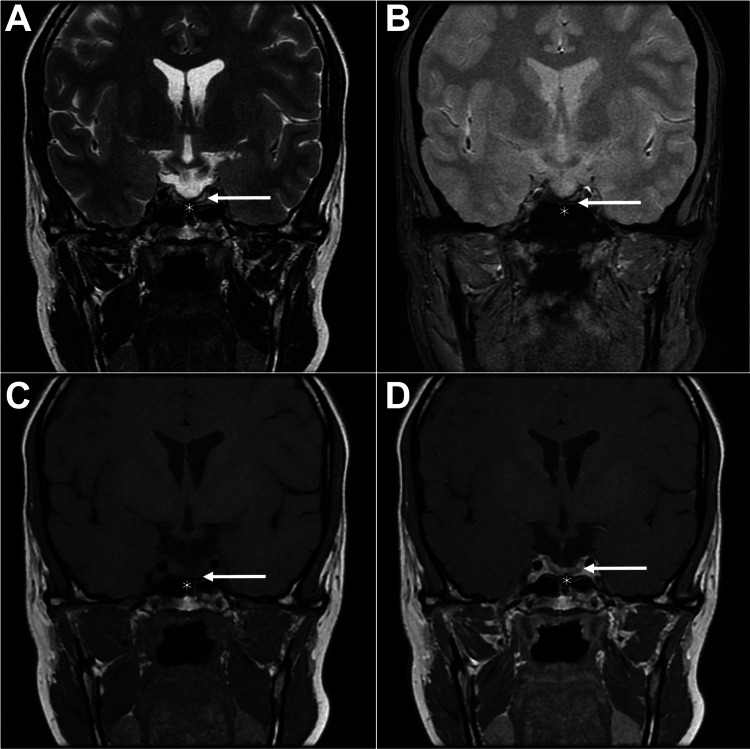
One-year postoperative follow-up MRI after transsphenoidal surgery Coronal T2-weighted (A), coronal T2*-weighted (B), coronal T1-weighted (C), and post-contrast coronal T1-weighted (D) images demonstrate postoperative changes consistent with a transsphenoidal approach, including sellar floor dehiscence (asterisk). The sella is filled with cerebrospinal fluid-equivalent signal intensity. A thin linear remnant of pituitary tissue is evident along the inferior margin (arrow). No residual tumor is identified. The optic chiasm appears normal.
